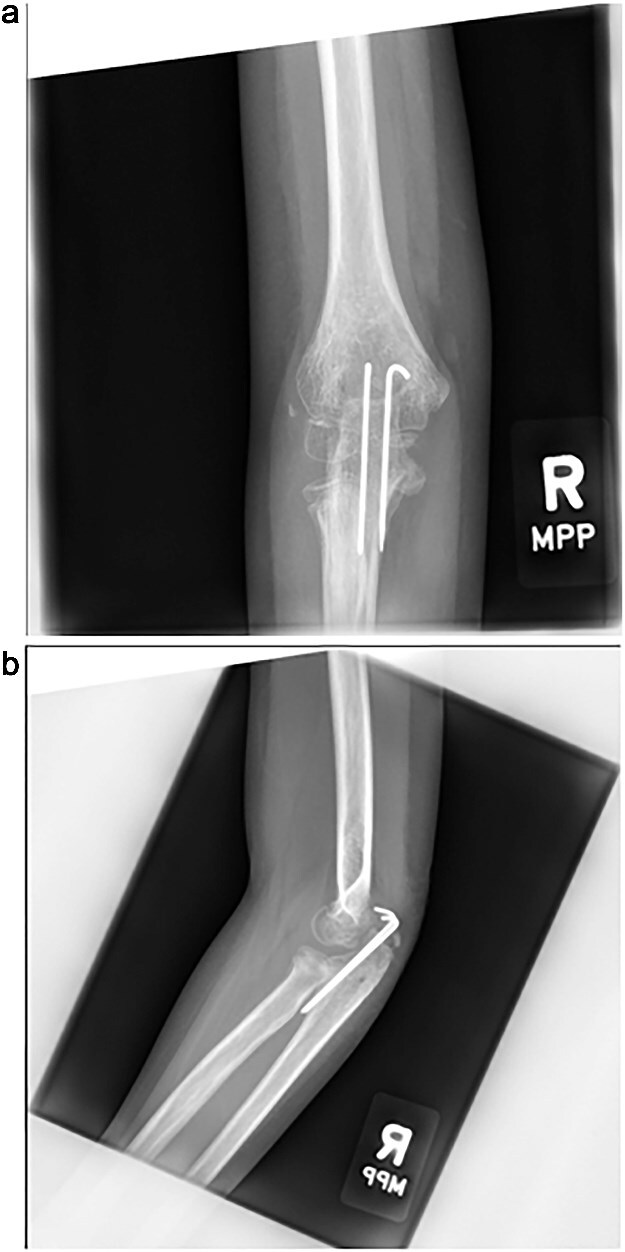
(a, b) Radiographs at 5 weeks, showing maintained alignment at the time of cast removal.
